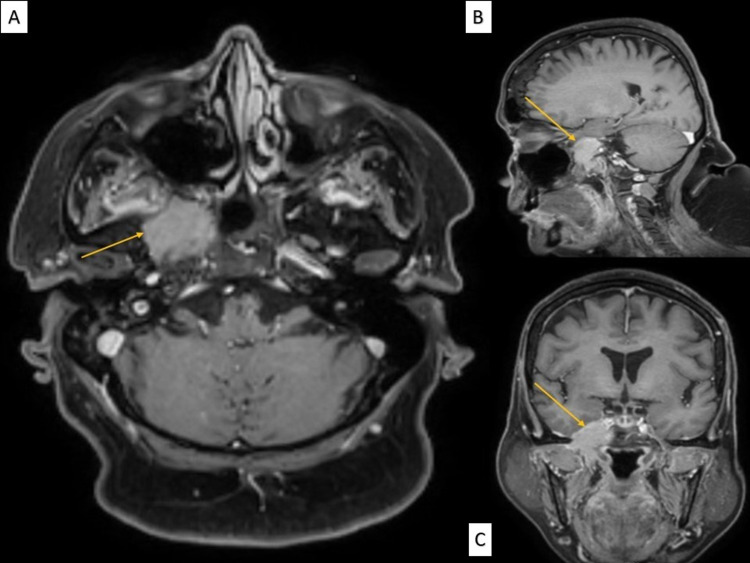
(A) Axial view, (B) sagittal view, and (C) coronal view of the MRI of the patient demonstrating a destructive solid heterogeneously enhancing mass of 2.9x2.4 cm (yellow arrow) centered in the medial portion of the right middle skull base involving the greater wing of sphenoid, lateral sphenoid body, and pterygoid plates extending into the intracranial portion and infratemporal fossa.
